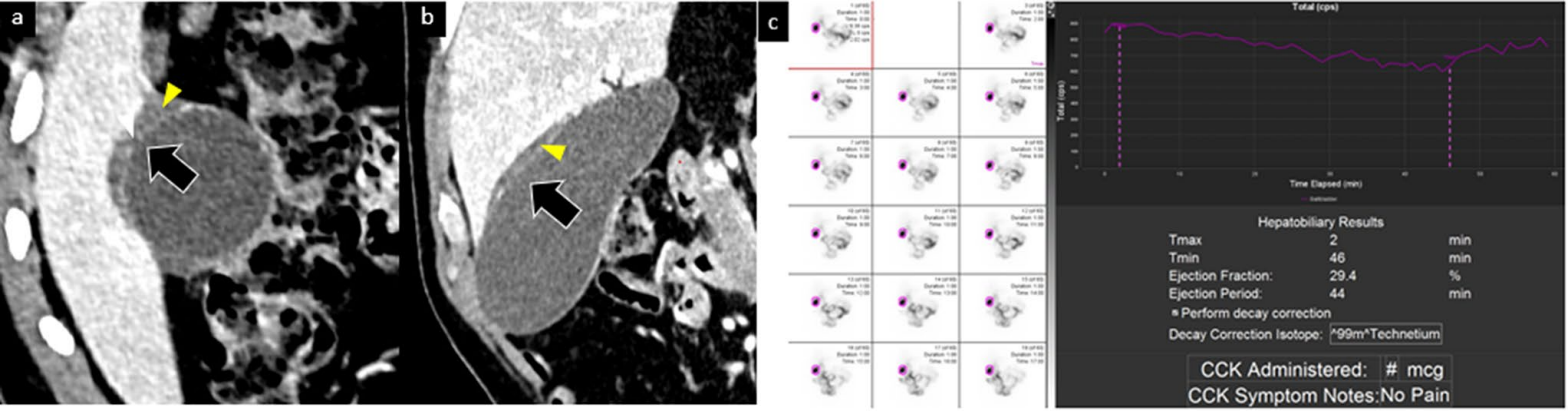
86-year-old man with over three months of right upper quadrant pain. Axial and coronal images of a contrast-enhanced CT (a, b) shows mildly increased gallbladder wall hyperenhancement with patchy areas hypoenhancement (black arrows) as well as a small amount of pericholecystic fluid. A follow up ultrasound did not show gallstones. A follow up nuclear medicine scintigraphy study (c) showed filling of the gallbladder but after a fatty meal a depressed ejection fraction of 29% was found (normal is greater than 33%), compatible with chronic cholecystitis
